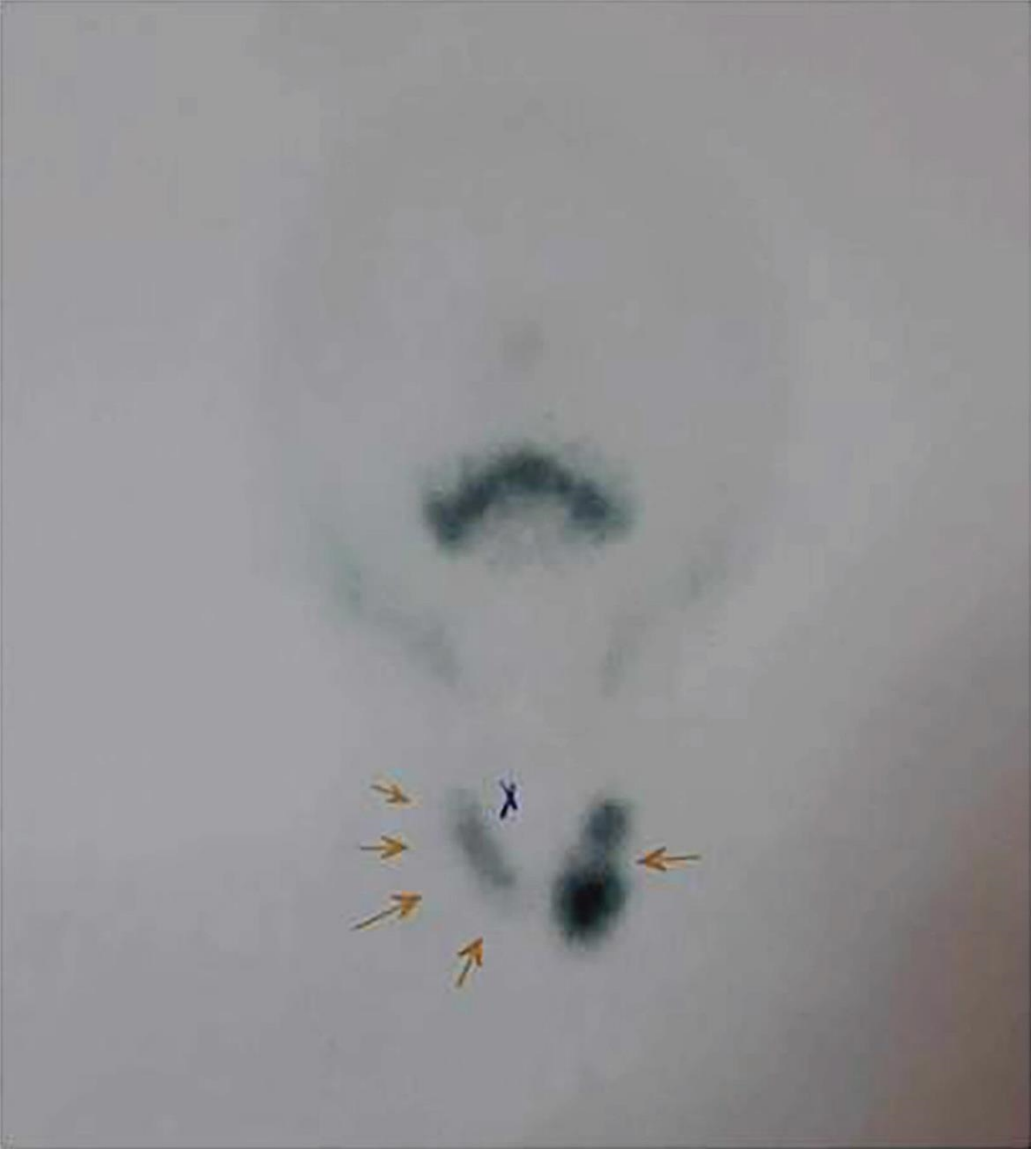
Thyroid scintigraphy with 99mTc‐pertechnetate showing suppression of the right lobe compressed by the adjacent parathyroid cyst, with a cold nodule in its upper third. The left lobe demonstrated a cold cystic nodule in the upper pole and a hot adenomatous lesion in the lower pole.
